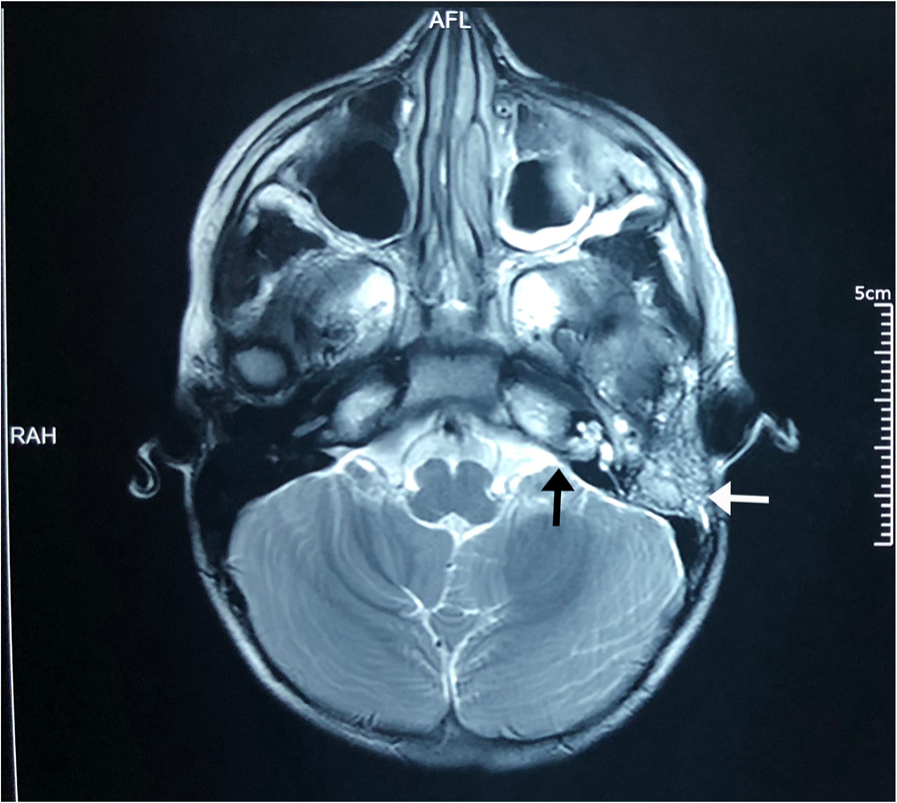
Contrast enhanced MRI brain demonstrating left mastoiditis; white arrow points to the mastoid sinus with mastoiditis and black arrow points to the petrous bone
